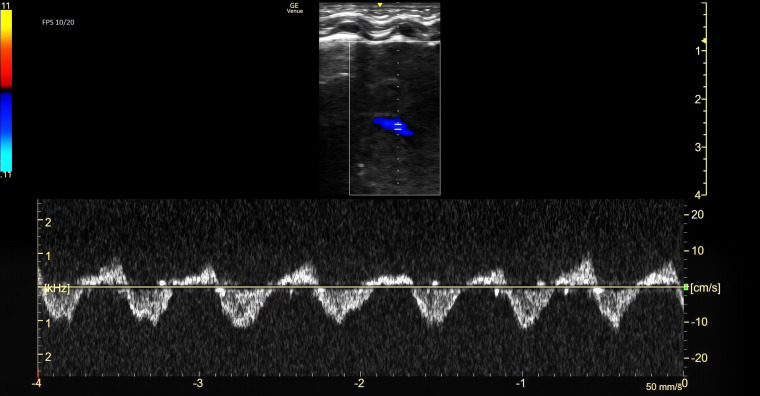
Point of care ultrasound (POCUS) of the right lung with color flow mapping and spectral Doppler demonstrating vascularity consistent with systemic venous flow.
